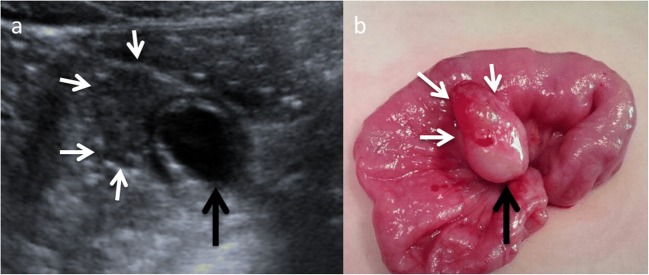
A 4-year-old boy in a routinary US control of a horseshoe kidney. a Abdominal ultrasound view of an EDC (*black arrow*) with a peripheral eccentric hypoechoic cap (*white arrows*). b Surgical findings: the gastric mucosa was visible as a polypoid mass (*white arrows*) arising from the external surface of the EDC (*black arrow*)
